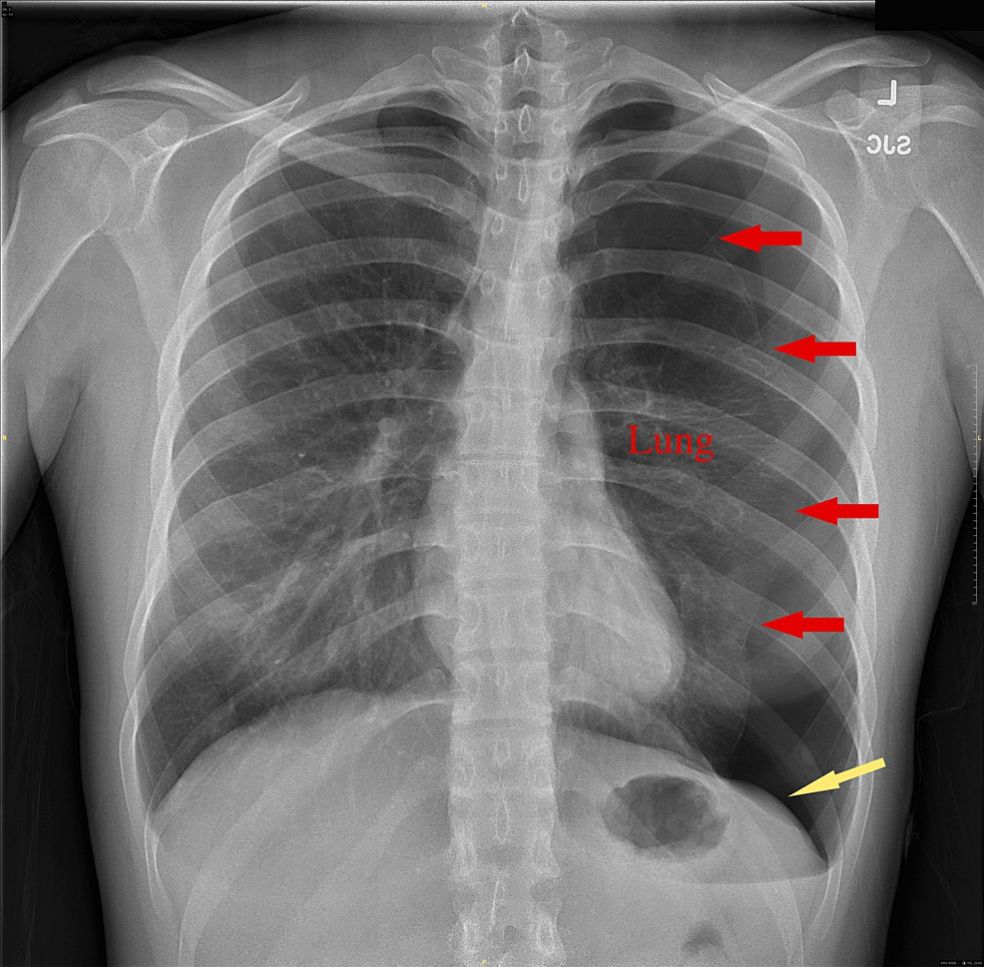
Chest x-Ray on presentation showing a left-sided pneumothorax, marked by red arrows. There is also a mild lowering of the left diaphragm (a deep sulcus sign, yellow arrow).
